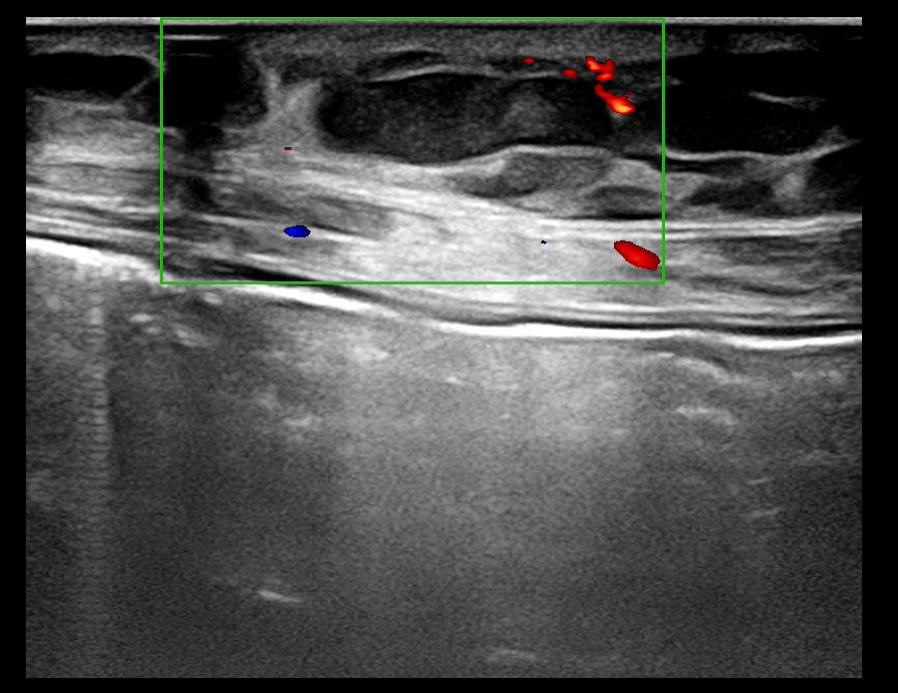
Ultrasound reveals multiple hypoechoic lesions in the abdominal subcutaneous tissue, some of which are confluent, with no blood flow signals detected.
